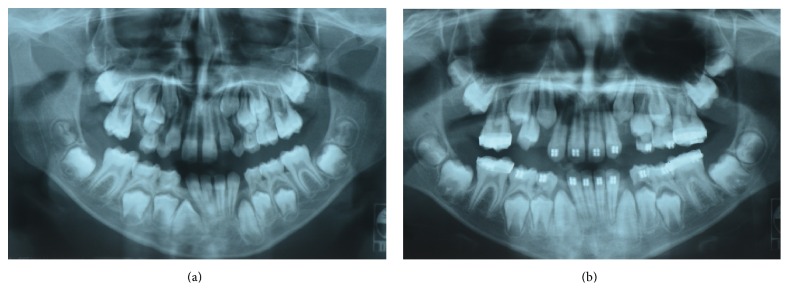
Panoramic radiographs of the patient aged (a) 9 years and 11 months and (b) 10 years and 4 months with no evidence of supernumerary teeth.
